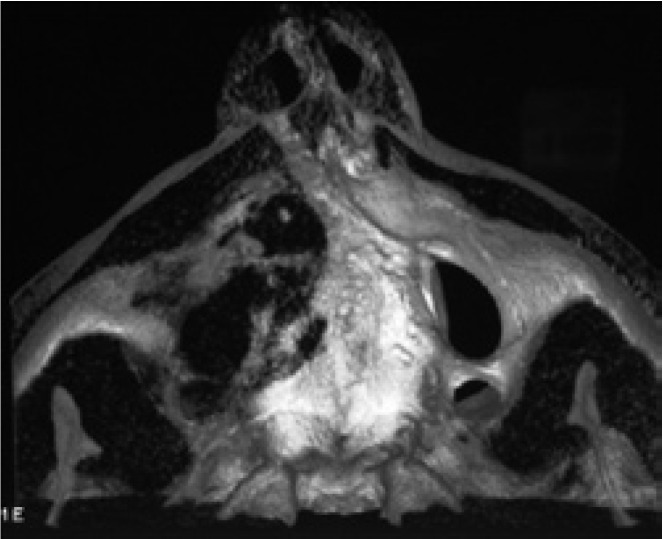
CBCT showing the borders of the lesion and the perforation areas.
